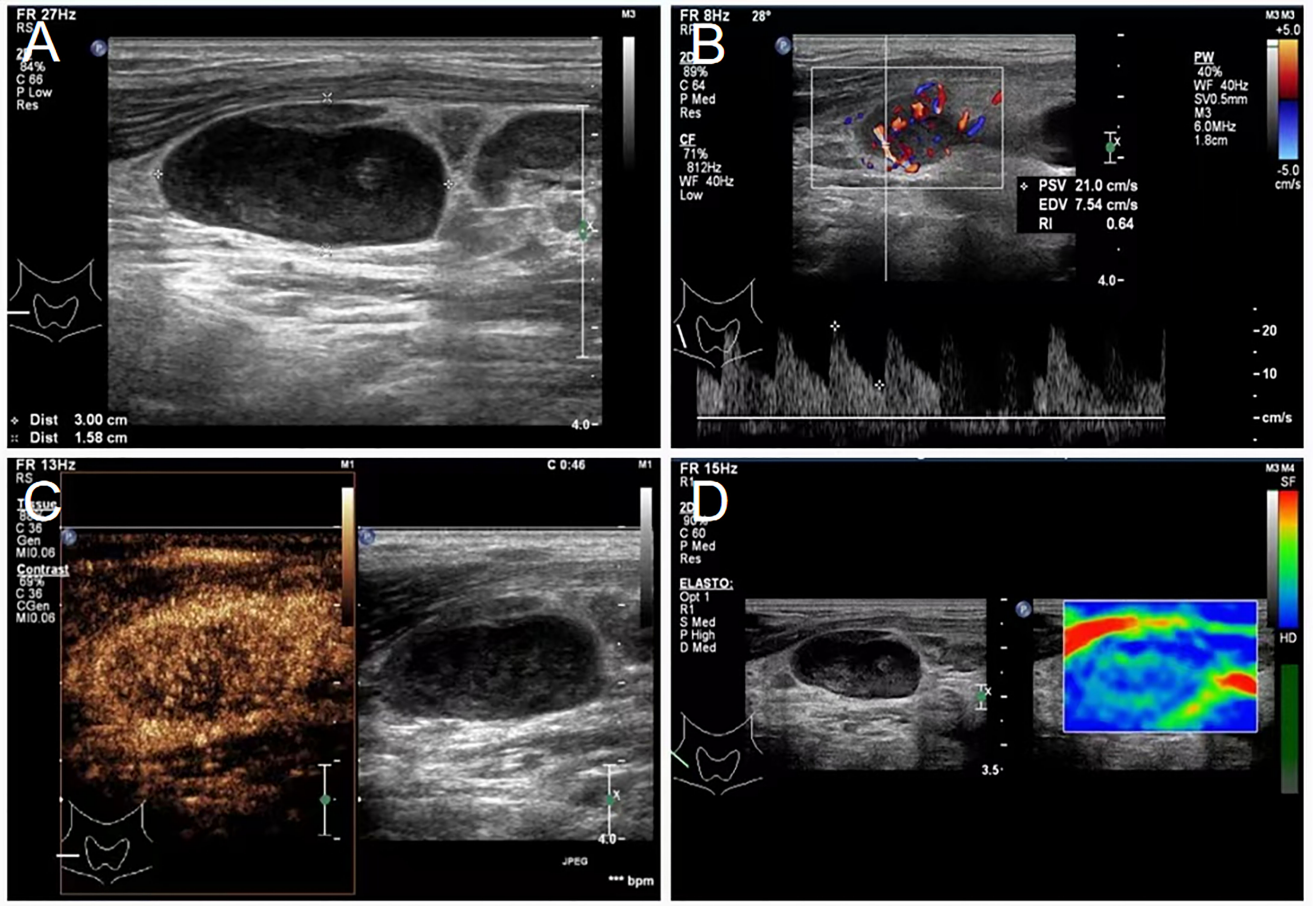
Lymphadenopathy noted on ultrasound in the neck of a 43-year-old male patient diagnosed with primary mediastinal B-cell lymphoma. (A) Sonogram revealing enlarged lymph nodes of the right neck, 3.0 × 1.6 cm in size, oval, uneven, and with a fair margin. (B) Color Doppler examination showing abundant blood flow in enlarged lymph nodes of the right neck, a lymphatic hilum, PSV: 21.0 cm/s, RI: 0.64. (C) Contrast-enhanced ultrasound showing abundant lymph node blood supply in area III of the right neck. (D) Shear-wave elastography of the lymph node, which is indicated by the mostly blue area. PSV, peak systolic velocity; RI, resistance index.
